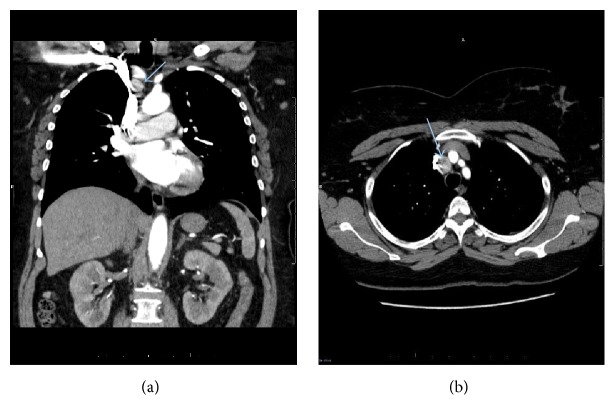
A right paratracheal mass in the upper mediastinum on the images of CTA from a patient presented with cough and right chest pain. A 61-year-old female presented to emergency room with cough and right chest pain. Chest CTA was performed to exclude pulmonary embolism as part of chest pain workup. One 2.0 × 1.7 cm right paratracheal mediastinal mass was visualized on the images of CTA, as indicated by a blue arrow on coronal (a) and axial (b) view images of CTA. This right paratracheal mass appeared hyperdense or showed mild contrast enhancement relative to other small mediastinal lymph nodes.
